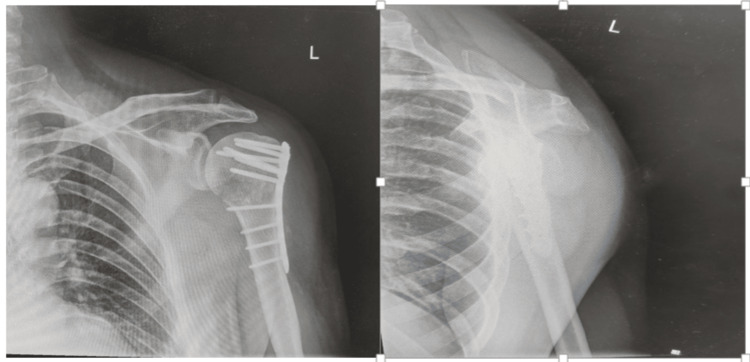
Anteroposterior and scapular Y view radiographs post open reduction internal fixation (ORIF) following a proximal humerus fracture Image Credits: First Author
